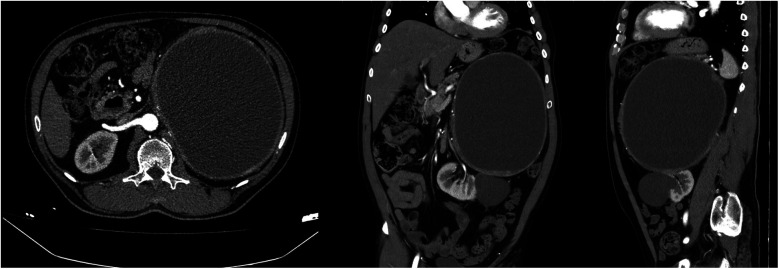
Computed tomography images of a giant left adrenal cystic mass and a left renal large parapelvic cyst.
